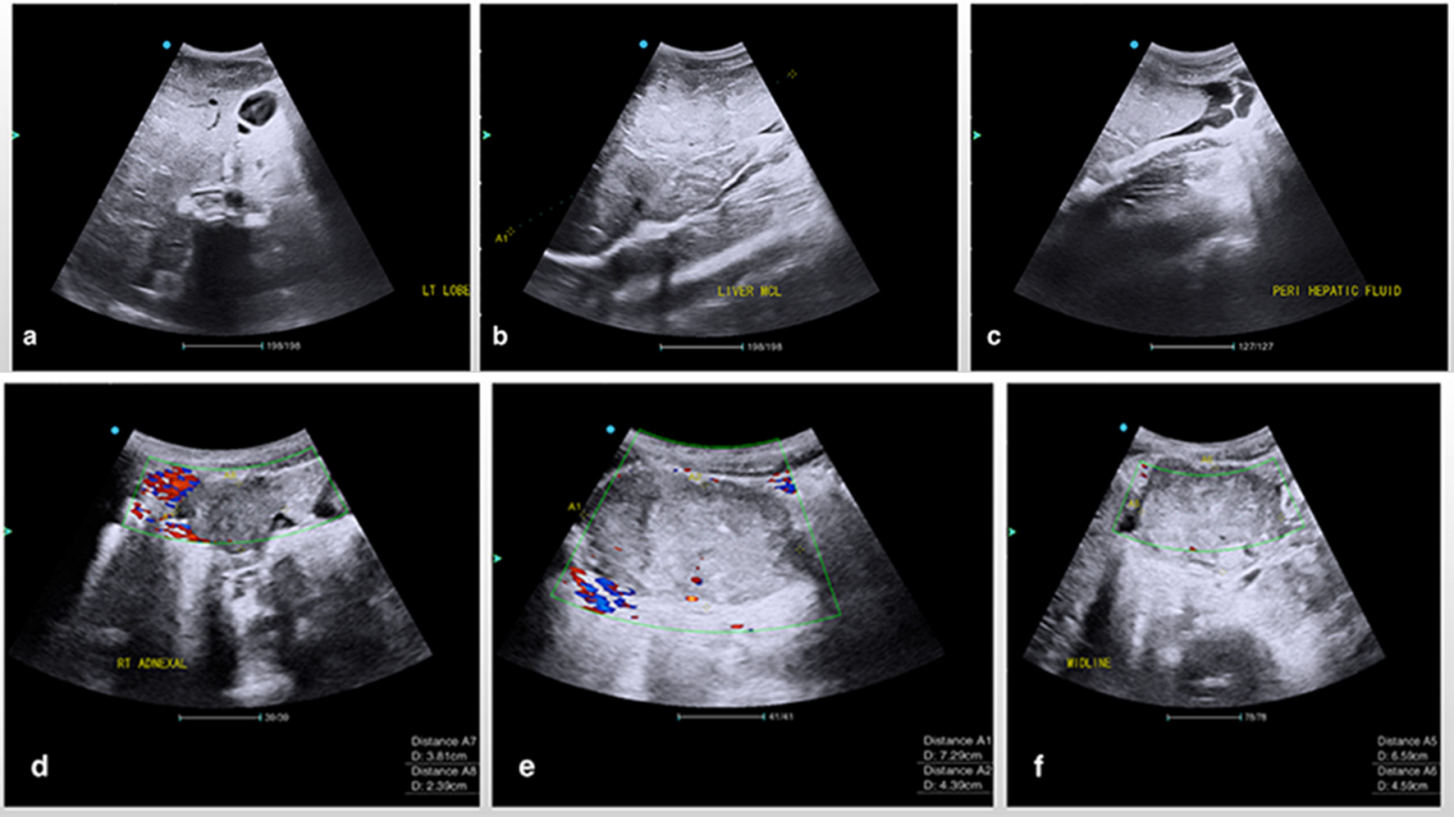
Abdominal ultrasound images in the sagittal and axial planes demonstrate the left and right lobes of the liver (b) with multiple isoechoic masses of varying sizes. Perihepatic free fluid consistent with ascites was also present (c) along with multiple heterogeneous hypovascular masses in the right adnexa (d), pelvis (e) and midline (f) regions.
